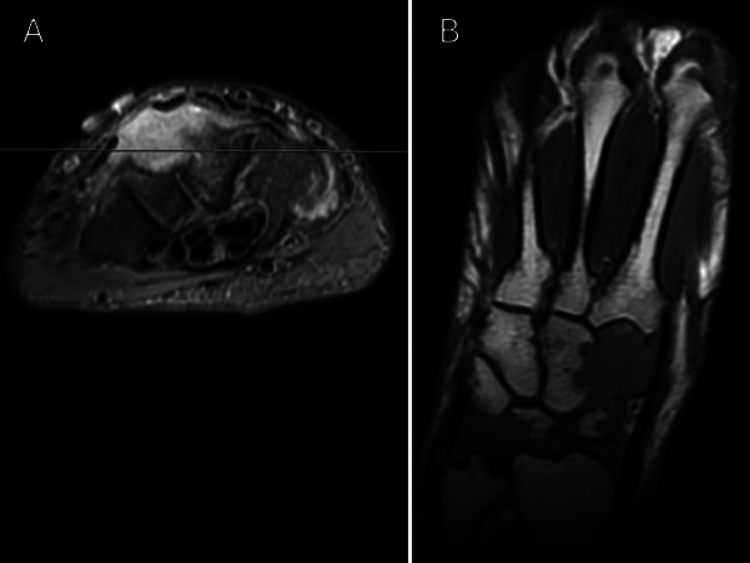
MRI of the right wrist with representative images of the dorsal wrist mass. (A) T2 hyperintense, heterogeneous mass deep to the ECRB and ECRL, appearing to erode into the capitate and trapezium. (B) T1 hypointense sequence re-demonstrating the mass eroding into the trapezium and capitate. ECRB: extensor carpi radialis brevis; ECRL: extensor carpi radialis longus
